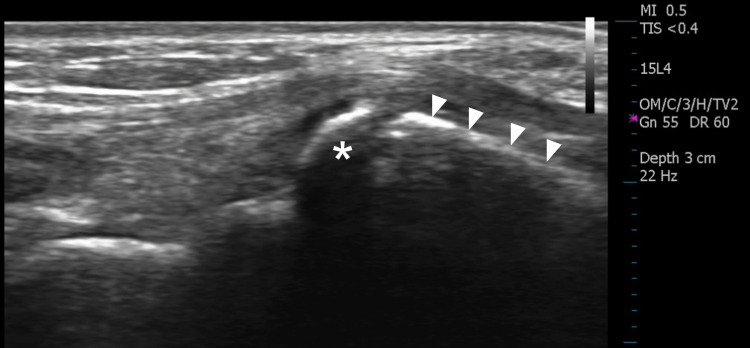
Longitudinal gray scale ultrasound view of the base of the symptomatic third metacarpal (arrowheads) demonstrating an accessory ossicle (asterisk). The left side of the image is proximal.
